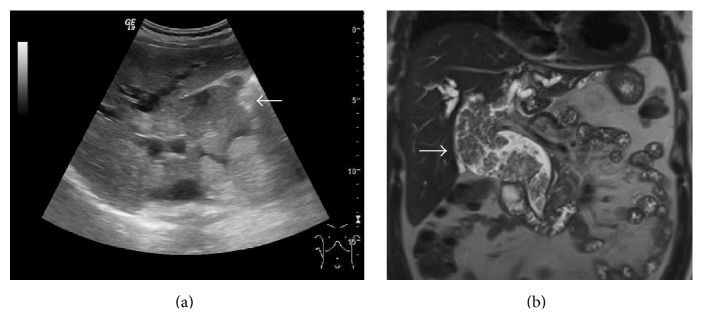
Preoperative imaging. (a) An ultrasonography and (b) MRCP revealed extensive papillary tumor in LHD, CHD, and CBD (arrow). LHD: left hepatic duct, CHD: common hepatic duct, and CBD: common bile duct.
